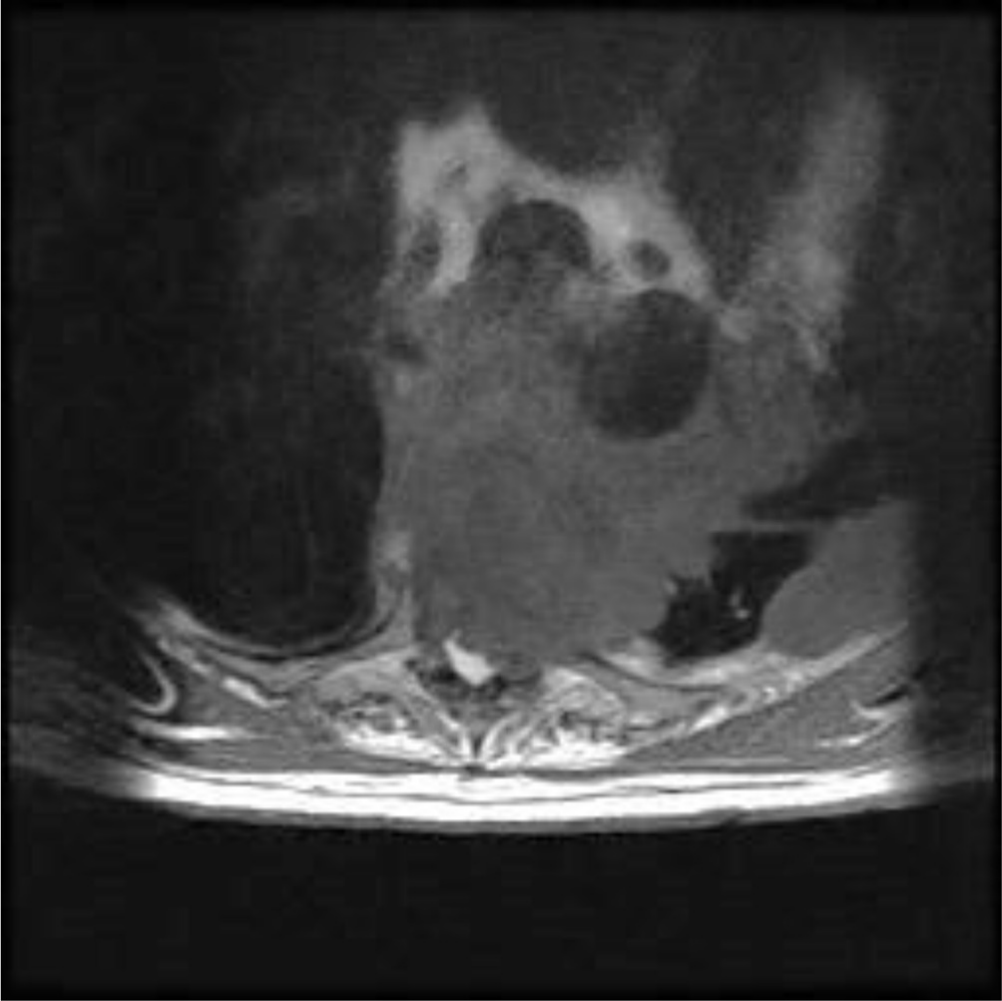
Axial slice of a CT scan showing left-sided lobulated pleural thickening extending into the lung as a 3-cm lobulated mass.
